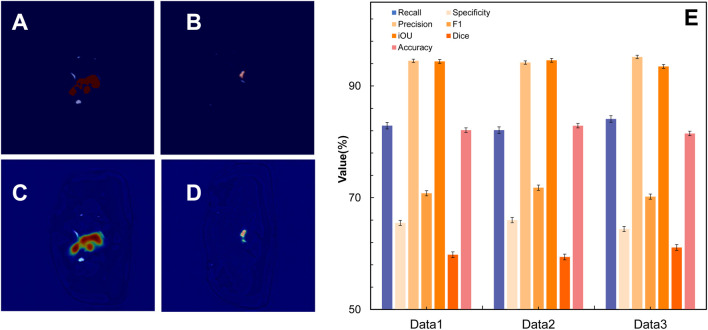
Segmentation performance of the CRFE model on cervical cancer MRI images. (A) Standard lesion contour of a certain MRI slice; (C) lesion contour obtained by the model after segmentation of the slice; (B) standard lesion area of all the other slices of the patient; (D) lesion contour obtained by the model after segmentation of the slice; (E) performance index of the CRFE model on three test sets of MRI images.
